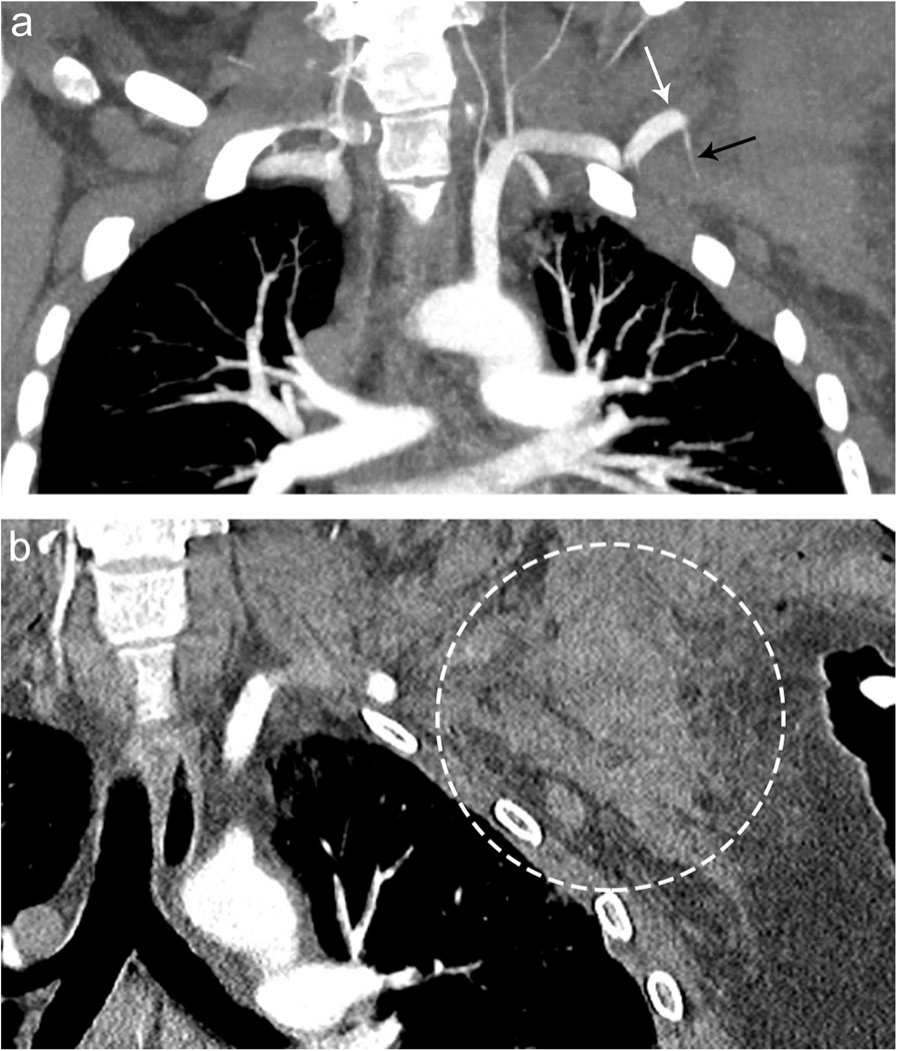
Transection of the left axillary artery. Patient presenting with multiple traumatic fractures and a cold left arm. Coronal (a) contrast CT image demonstrates abrupt cutoff of the left axillary artery (white arrow), along with active extravasation of contrast material (black arrow). Coronal (b) contrasted CT images demonstrate associated hematoma (dashed circle) in this corresponding region
